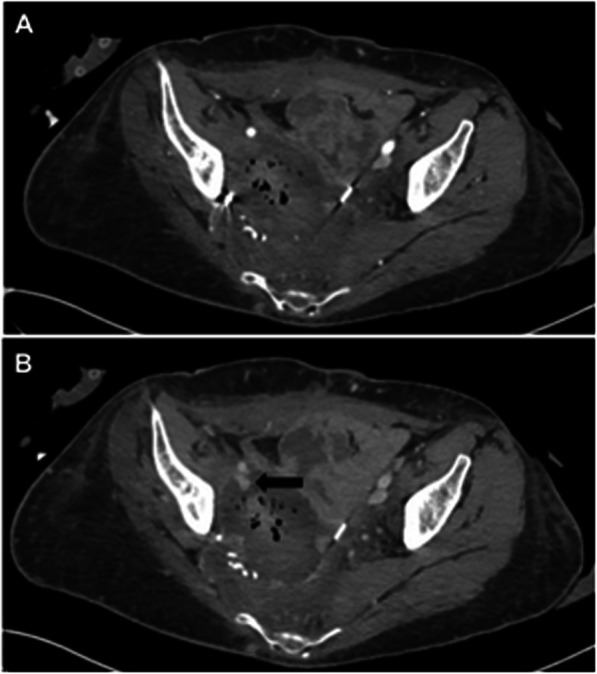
Arterial (A) and venous (B) phase imaging of CT-abdomen/pelvis demonstrates interval development of venous phase hyperattenuation in the chronic pelvic collection with locules of air, adjacent to a new sac-like dilation arising from the right EIV (black arrow), concerning for vascular erosion by the chronic pelvic abscess
